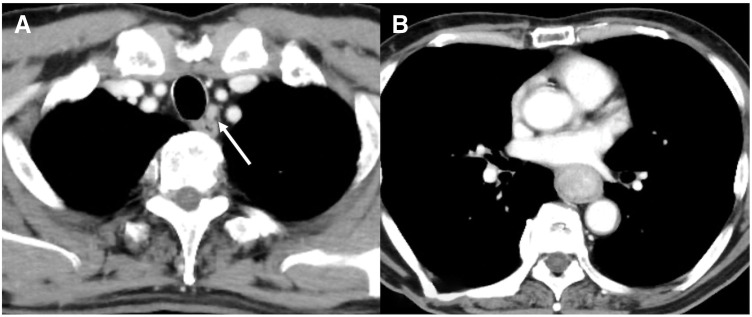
Contrast-enhanced CT image. (A) Swelling of the left upper mediastinal lymph node (arrow). (B) The tumor bordering the pericardial sac in the mid-thoracic esophagus.
